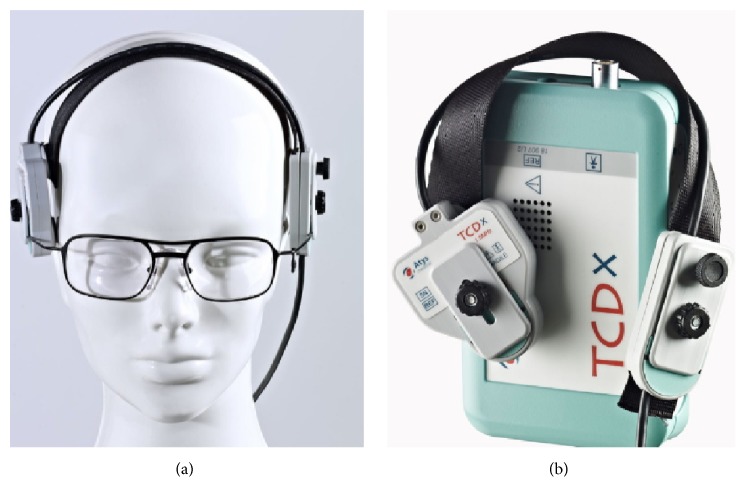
(a) Robot probe and (b) Holter Transcranial Doppler System (TCD-X, Atys Medical, Soucieu en Jarrest, France).
